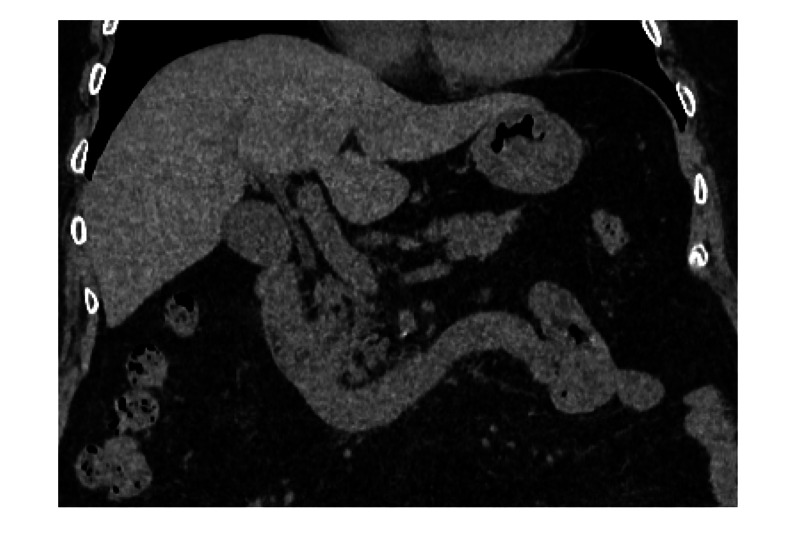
The abdominal computed tomography findings obtained the next day It shows no evidence of perforation, and the ileus had disappeared.
